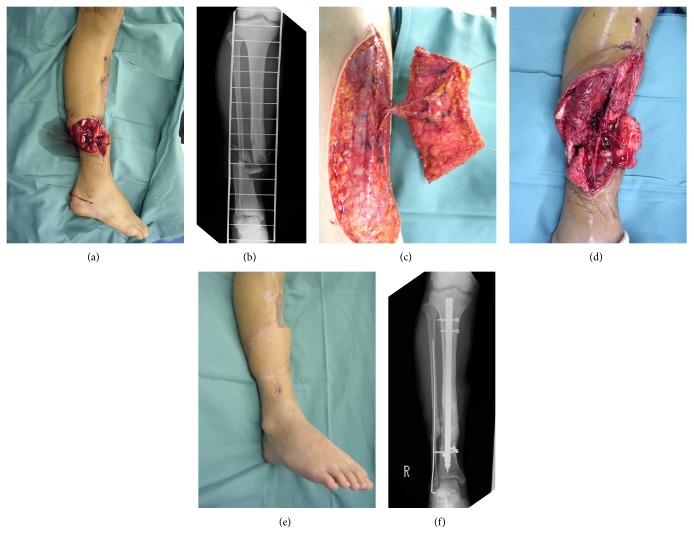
(a) Open fracture is located in the distal third of the lower extremity, accompanied by injury to the anterior tibial artery. (b) X-ray findings. The open fracture is accompanied by a bone defect. (c) The flow-through type anterolateral thigh flap harvested from the same side. (d) The anterior tibial artery is reconstructed by flow-through anastomosis with the lateral circumflex femoral artery. (e) Appearance at 18 months postoperatively. (f) X-ray findings at 18 months postoperatively, showing that union of the bone defect occurred after autologous bone grafting.
